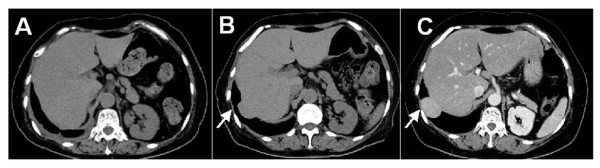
CT findings of the tumor. The mass in the right lung field with its inferior border abutting the diaphragm (arrow) increased in size over time. A At the first admission. B At 3 months, and C 12 months after the operation for lung cancer.
